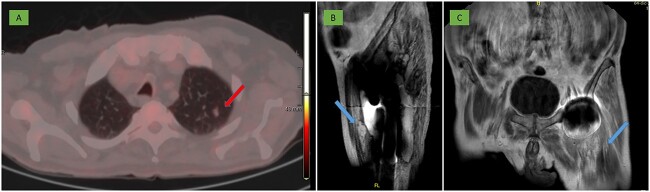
(A) PET-CT: 10 mm nodule in the left pulmonary apex with incipient metabolic activity suggestive of malignancy (arrow). (B, C) MRI: deep residual lesional focus in the anterolateral region dependent on the anterior rectus muscle (arrow).
